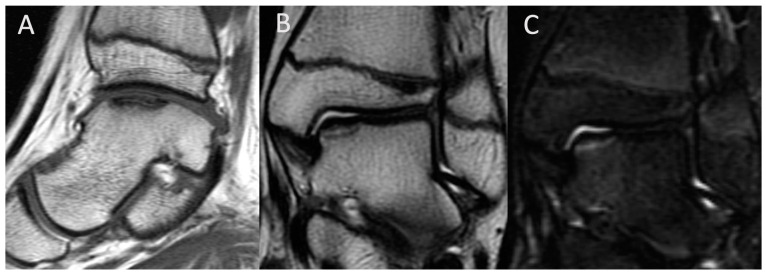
MRI image of talus OCD. (A) T1-weighted image (sagittal view); (B) T2-weighted image (coronal view); (C) short T1 inversion recovery (STIR) image (coronal view).
